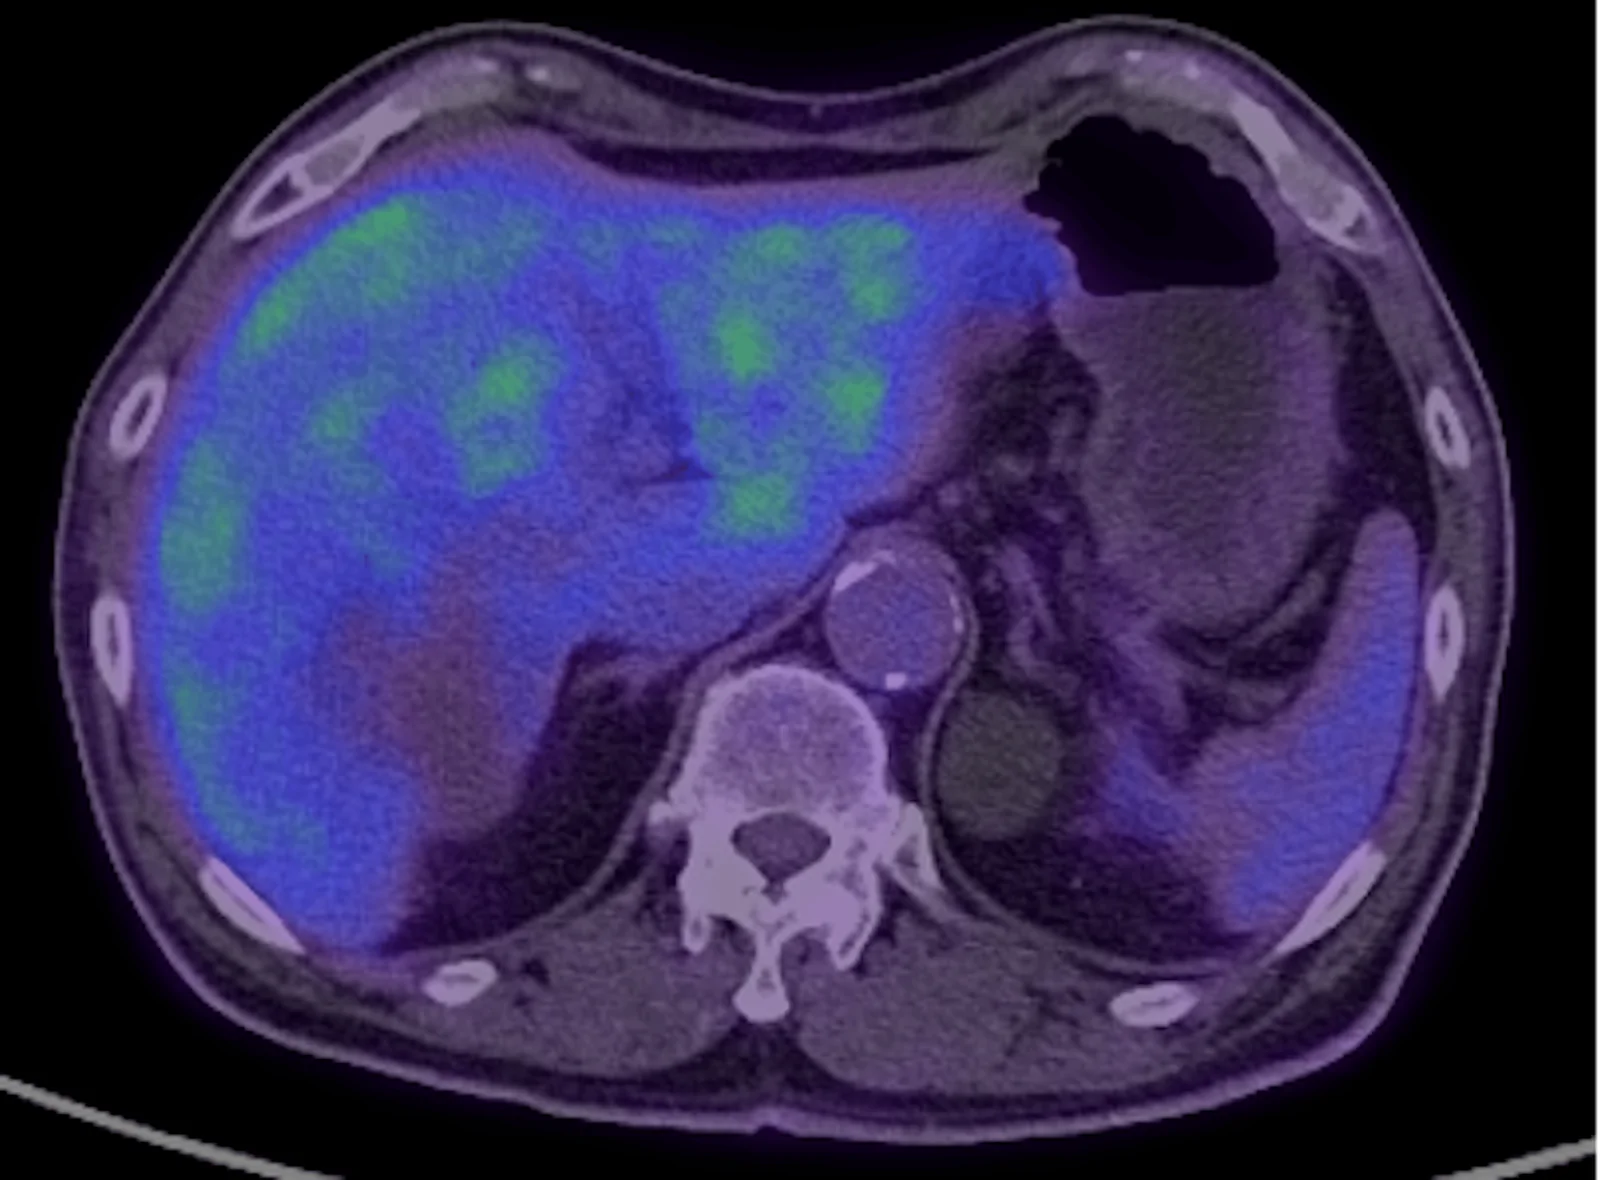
Axial positron emission tomography/computed tomography (PET/CT) image of the upper abdomen after six cycles of pembrolizumab and before hepatic resection PET/CT performed after six courses of pembrolizumab and before hepatic resection demonstrating markedly decreased or absent FDG uptake in the hepatic lesions, suggestive of post-treatment changes and diminished tumor metabolic activity.
